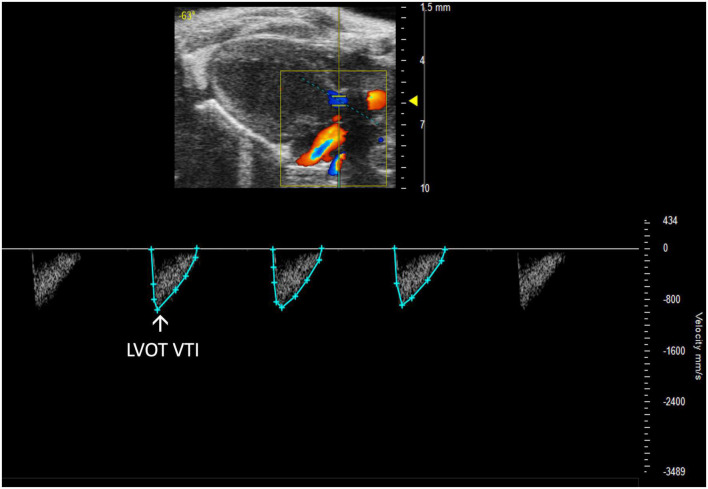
Representative image displaying left ventricular outflow tract velocity time integral (LVOT VTI), a measure of blood flow out of the ascending aorta which is obtained by calculating the area under the curve in the PW Doppler mode of the parasternal long axis view.
